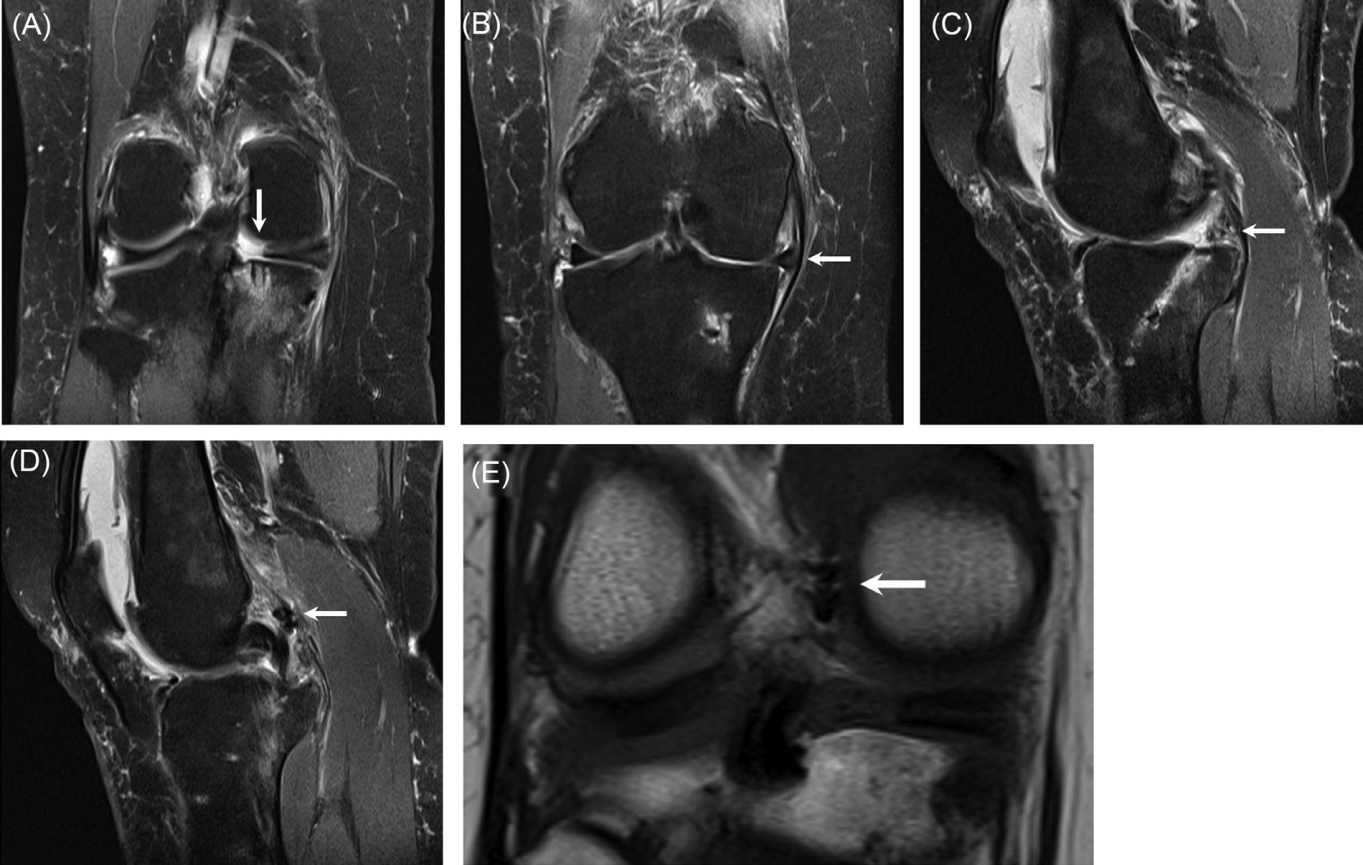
(A) Postoperative MRI. Coronal PD FS MRI image shows discontinuity of the posterior root of the medial meniscus at the level of the transtibial tunnel, consistent with tear (arrow). (B) Postoperative MRI. Coronal PD FS MRI image shows increased extrusion of the body of the medial meniscus from the joint space (compared to Fig. 1B), with bowing of the overlying medial collateral ligament (arrow). (C) Postoperative MRI. Sagittal PD FS MRI image shows abnormal signal and morphology of the posterior root of the medial meniscus (arrow) which does not appear continuous with the transtibial tunnel. The transtibial tunnel extends obliquely from posterosuperior to anteroinferior in the proximal tibia. (D) Postoperative MRI. Sagittal PD FS MRI image shows a focus of susceptibility artifact posterior to the intercondylar notch, likely representing a displaced suture (arrow). This is posterior to the posterior cruciate ligament. (E) Postoperative MRI. Coronal T1 MRI image shows a hypointense structure projecting in the posterior aspect of the intercondylar notch, likely representing a displaced suture (arrow).
